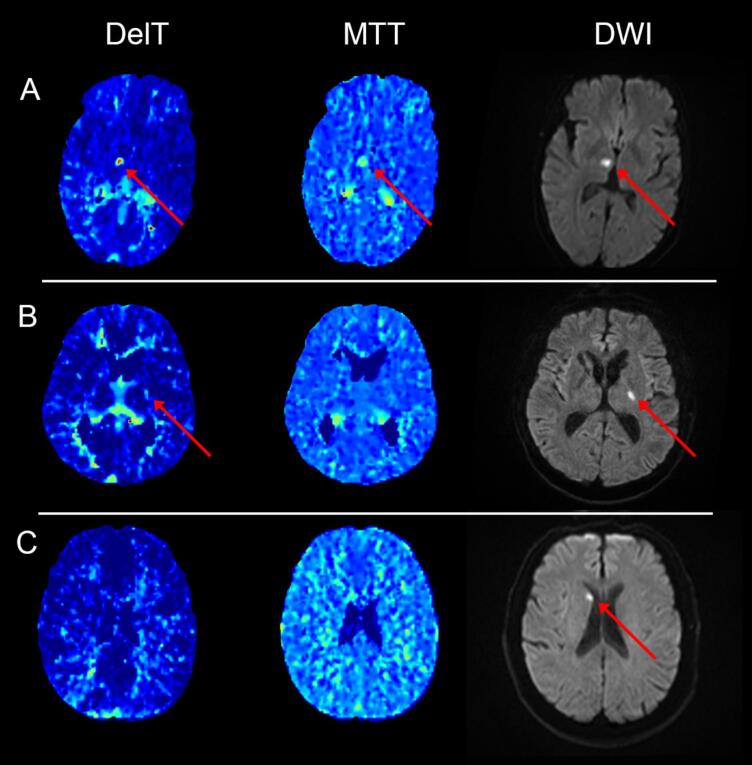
CTP Maps co-registered to DWI (A) – Obvious CTP lesion, (B) – Subtle CTP lesion, (c) – No CTP lesion. Lesion is highlighted in red across modalities. (For interpretation of the references to colour in this figure legend, the reader is referred to the web version of this article.)
